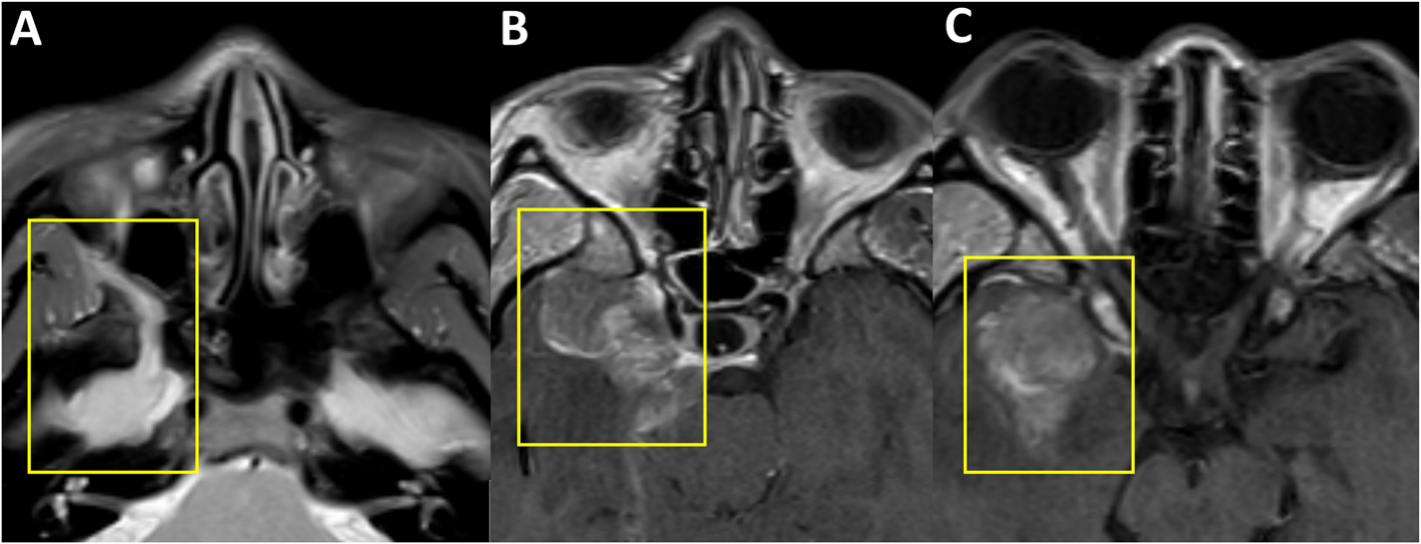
Radiographic Images of the Primary Sinonasal Carcinoma with perineural extension. Magnetic resonance images in the axial planes are presented. Preoperative images demonstrate a right sided mass along the trigeminal nerve (a). Following adjuvant radiation, the patient had progression with extension of tumor into the cavernous sinus (b) and an associated intradural metastasis (c). Yellow boxes highlight the tumor region of interest
